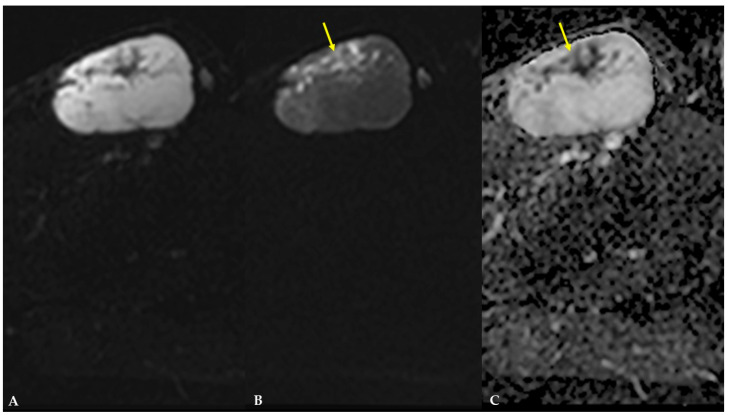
DWI and ADC images of MLS demonstrating signs of restricted diffusion within the mass (arrow): (A) b value = 0; (B) b value = 1000; (C) ADC map with measured ADC value of 0.8 × 10^−3^ mm^2^/s within the non-fatty non-myxoid portion and 2.5 × 10^−3^ mm^2^/s within the myxoid portion of the mass (not shown on image).
